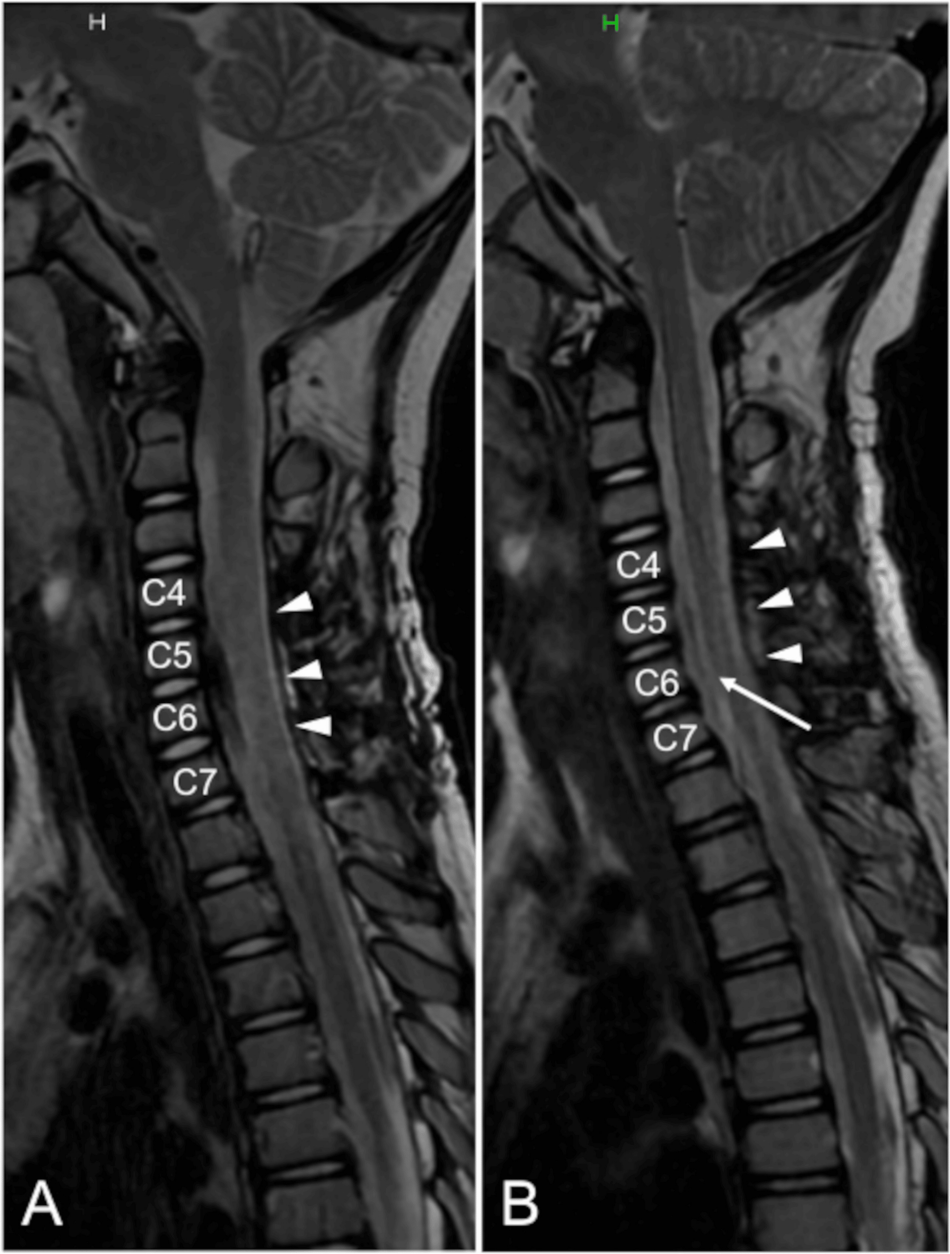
Postoperative cervical magnetic resonance images The compression of the spinal cord was resolved postoperatively (white arrowheads) (A). Any recurrence of the cyst was not observed two years after surgery (white arrow) (B) (sagittal T2-weighted images, A: one week after surgery and B: two years after operation).
